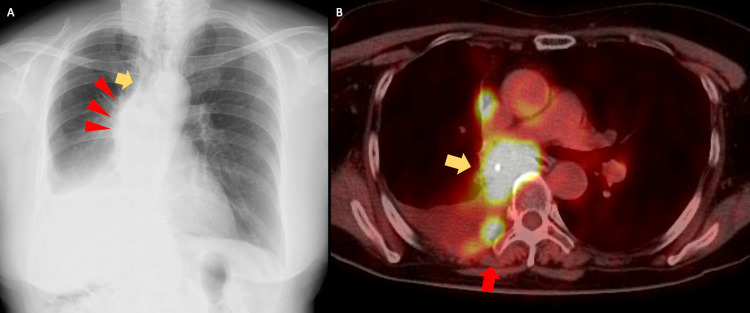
(A) Chest X-ray at the time of the initial examination. (B) PET-CT image at the time of the initial examination. (A) Chest X-ray showed thickening of the right paratracheal line (yellow arrow) and protrusion of the right first arch (red arrowhead) and decreased permeability of the right lower lung field due to pleural effusion. (B) PET-CT revealed a mass near the end of the right lower lobe bronchus postoperatively (yellow arrow), with strong 18F-labeled fluorodeoxyglucose accumulation in the same area. In addition, right pleural dissemination, eighth and ninth rib metastasis (red arrow), and multiple mediastinal lymph node metastasis were observed. PET-CT, positron emission tomography-computed tomography
